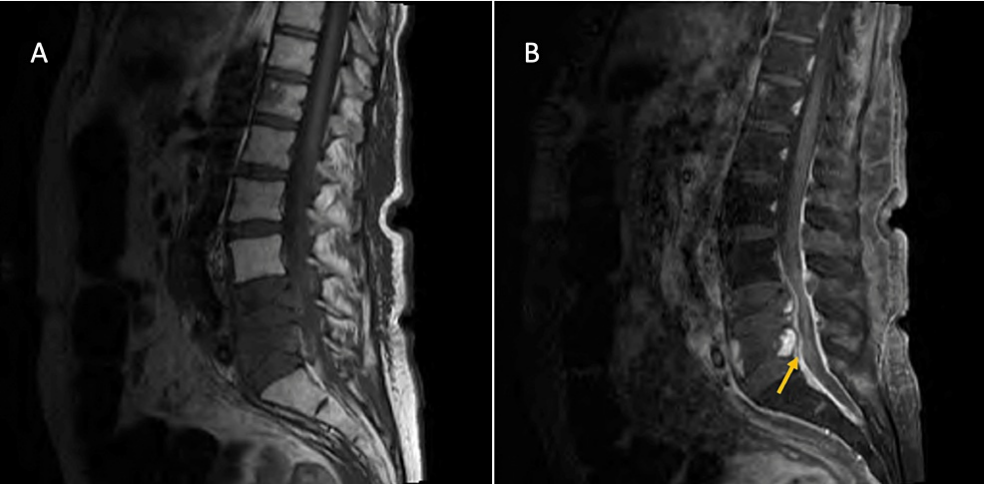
Magnetic resonance imaging of lumbar spine Lumbar magnetic resonance imaging (MRI) sagittal section, T1 sequence (A) without and (B) with gadolinium contrast, showing compression fracture at L4 and L5 vertebrae with contrast-enhancing mass and retropulsion and dural enhancement (arrow).
